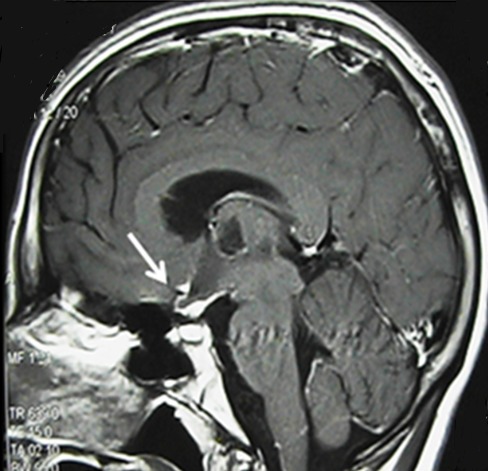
Cerebral MRI sagittal T1 weighted image: hypothalamic enhancement after contrast administration
